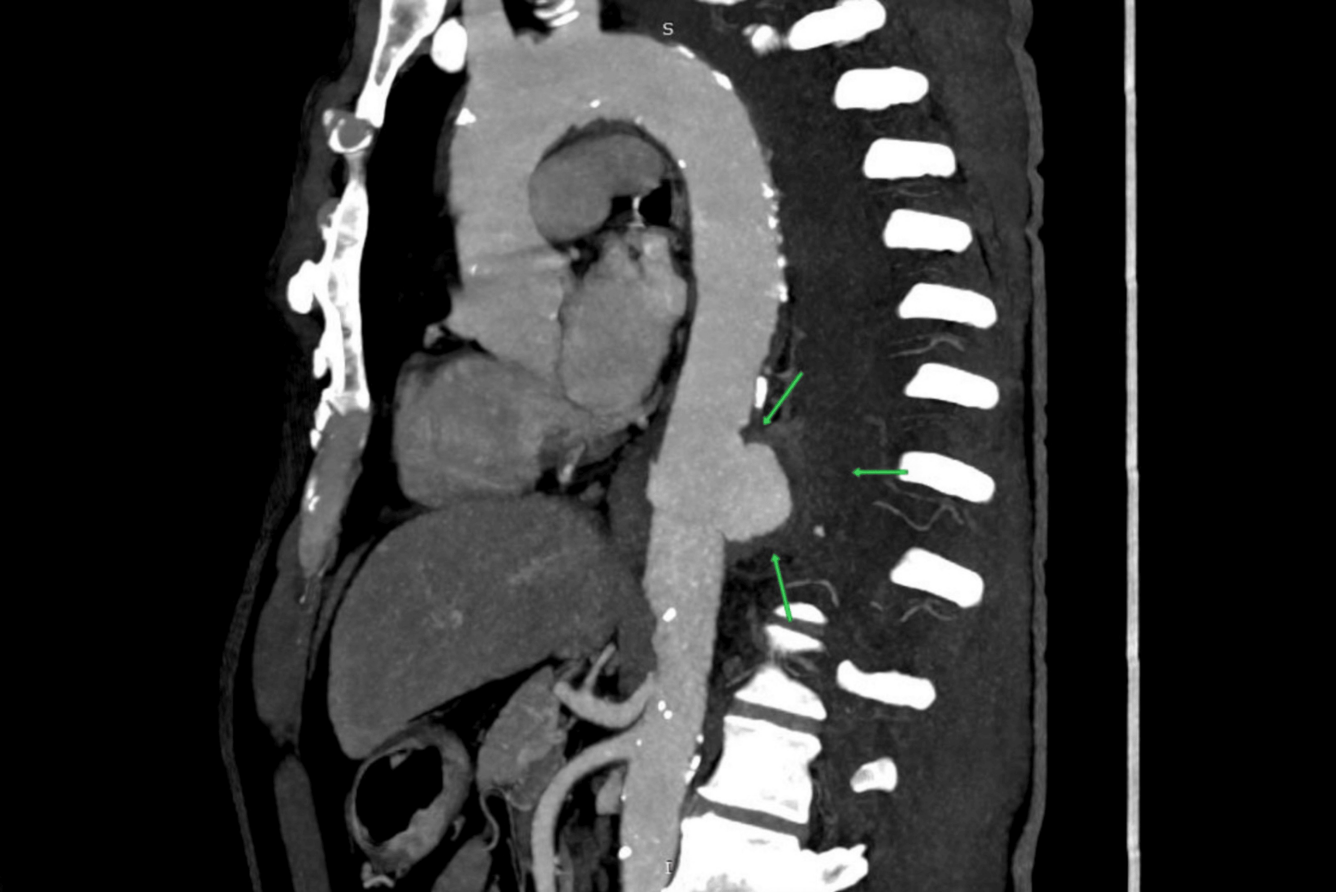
Computed tomography angiography (CTA) showing a saccular aneurysm of the descending thoracic aorta with a contained rupture, as indicated by the arrows.
